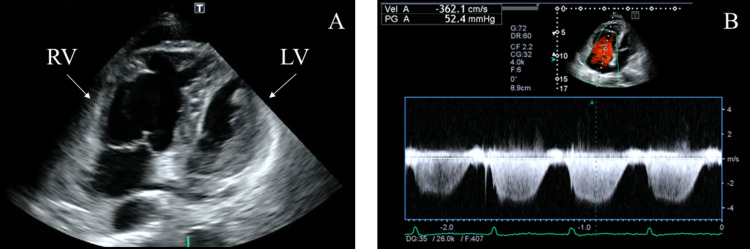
Transthoracic echocardiography on admission. (A) Transthoracic echocardiography showed right ventricular (RV) dilatation, flattening of the interventricular septum, and akinesia of the RV mid-free wall except for the apex, known as McConnell’s sign. (B) Tricuspid regurgitation with a pressure gradient (TRPG) was elevated at 52.4 mmHg.
